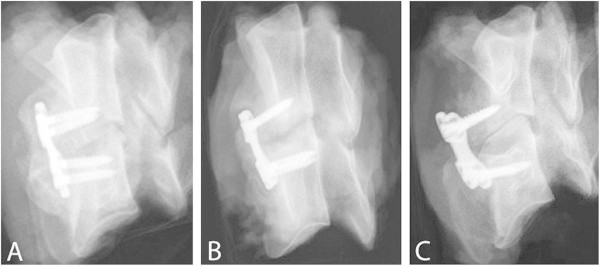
Radiographic follow-up. A Radiologic evidence of solid arthrodesis with complete bone bridging anterior to the motion segment and solid interbody fusion after 24 weeks, RS 2 (magnesium-PCL implant C3/4), but also with persistent evidence of the skeletal structure of the magnesium-PCL cage. B Radiolucent discontinuity in the interbody fusion mass and incomplete bone bridging anterior to the motion segment after 12 weeks, indicating RS 1 (bone graft C5/6). C No signs of interbody fusion after 6 weeks, RS 0 (bone graft C3/4).
